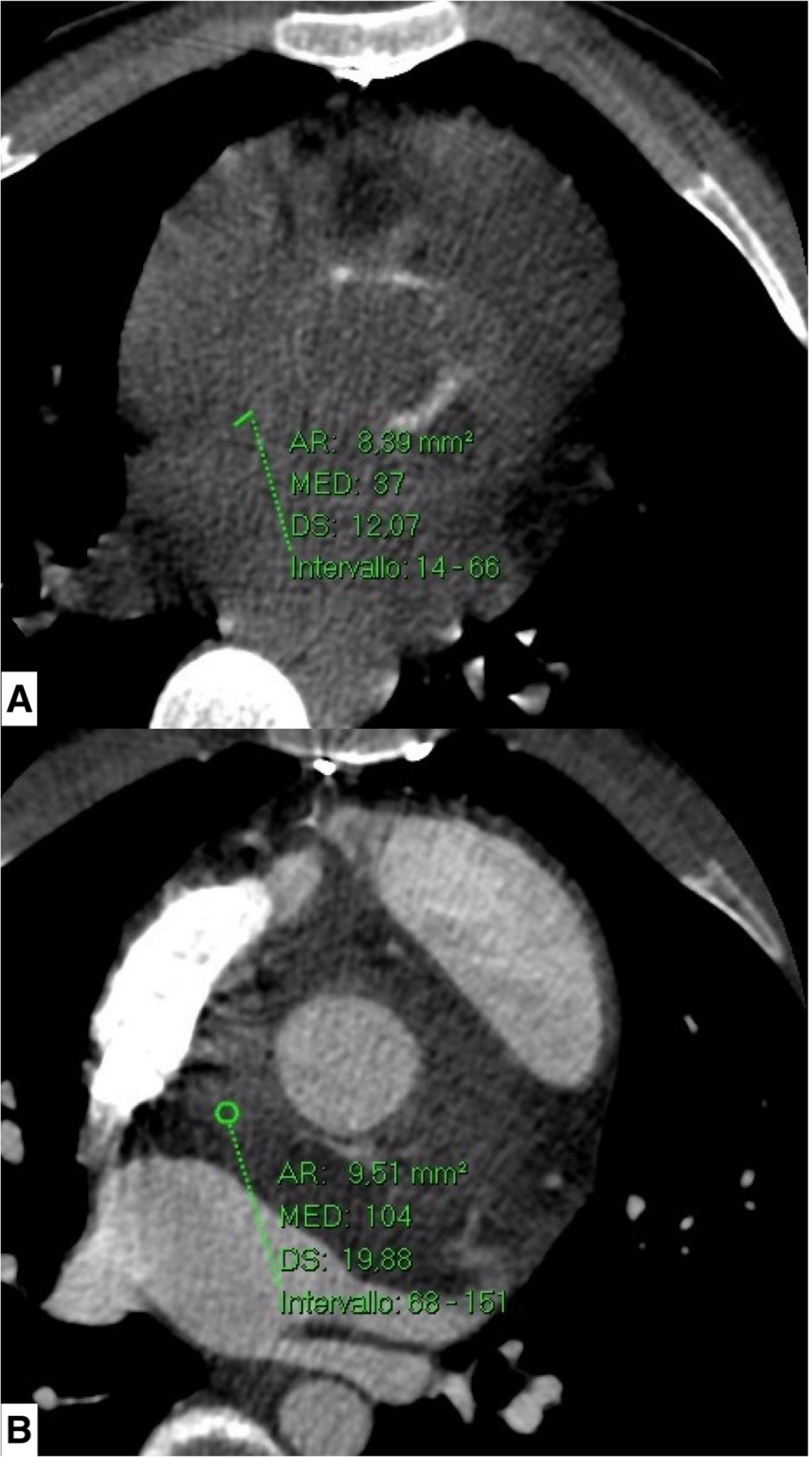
Computed tomographic scan. Hypodense area in the basal scan (a) in the periprosthetic site at the level of the anterior mediastinum, not characterized by significant contrast enhancement in the only arterial acquisition phase (b) carried out (HU (Hounsfield Unit) 37 without contrast medium; HU (Hounsfield Unit) 104 in arterial phase)
